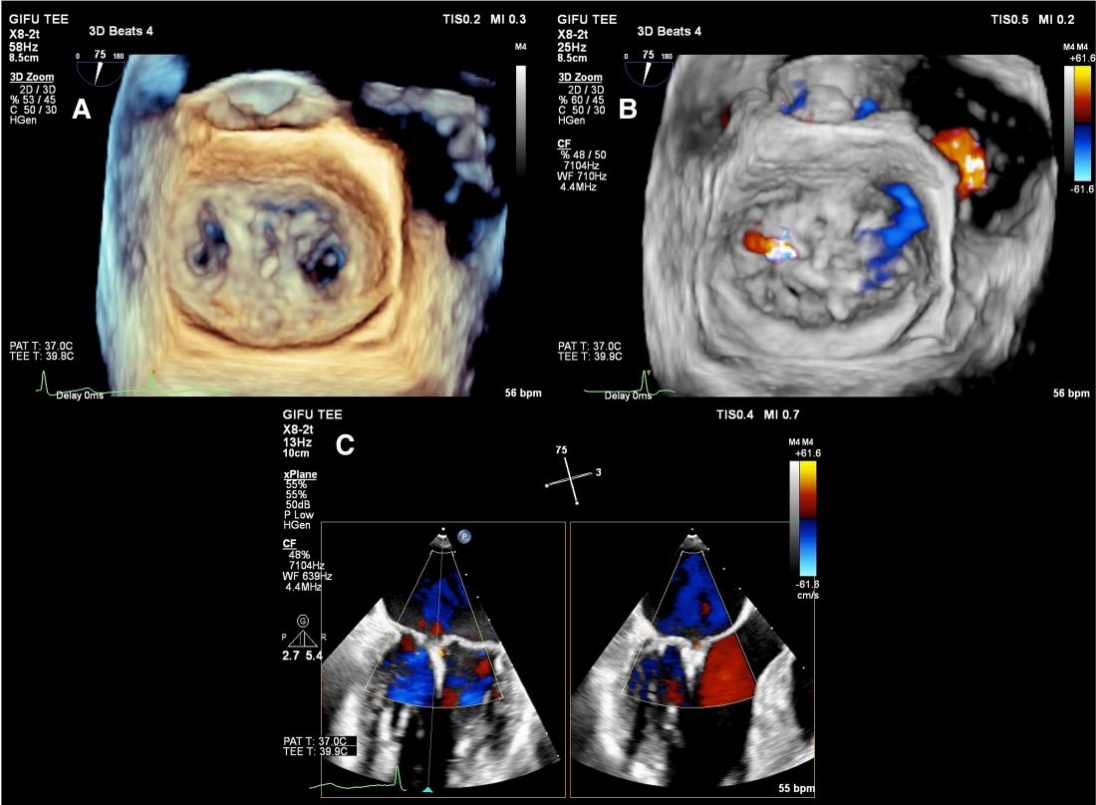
Intraoperative transoesophageal echocardiography during mitral valve transcatheter edge-to-edge repair. (*A*) 3D view: two MitraClips in place. (*B*, *C*) Colour Doppler: residual trivial mitral regurgitation.
